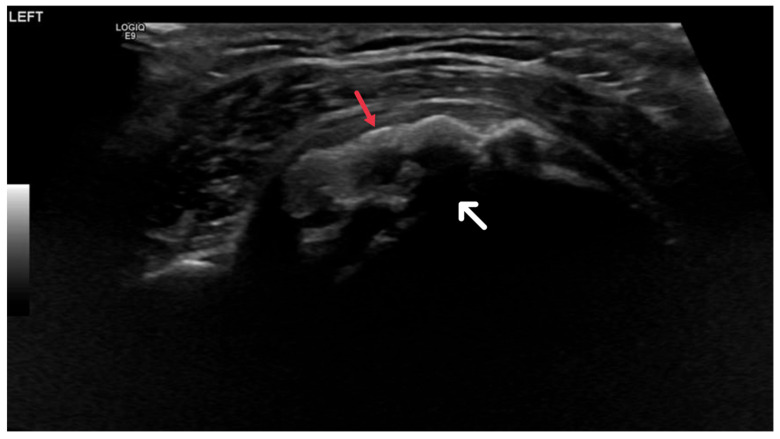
Ultrasound image showing calcific focus at the supraspinatus tendon insertion (red arrow) with posterior acoustic shadowing (white arrow).
